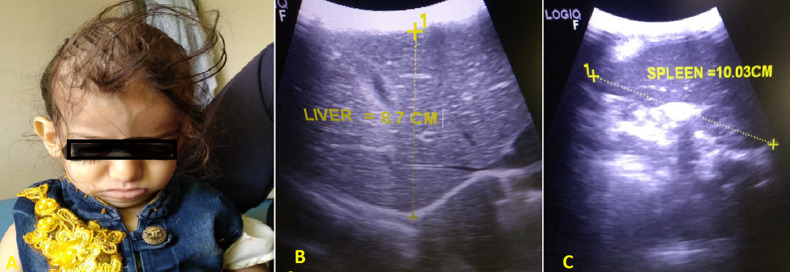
A) frontal bossing; ultrasonography image showing; B) hepatomegaly; C) splenomegaly
